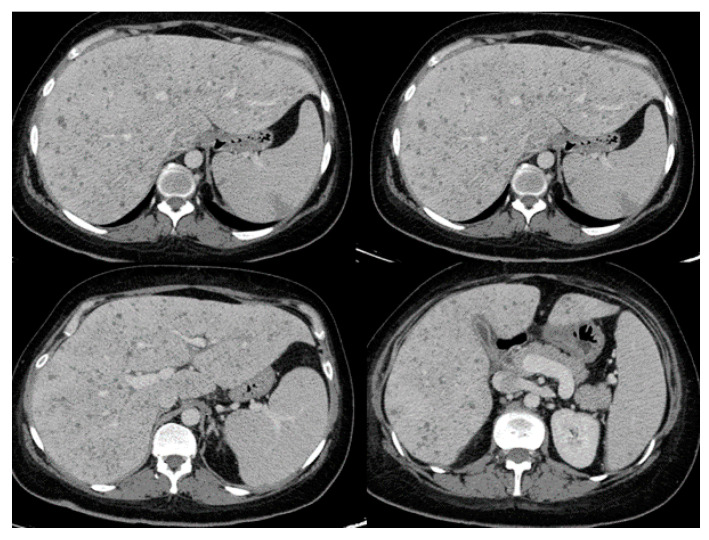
Abdominal computed tomography showing hepatosplenic abscesses in a patient with invasive *Saprochaete clavata* infection (reproduced from Del Principe, M.I et al. Mycoses 2016, 59, 594–601, doi:10.1111/myc.12508 with permission from John Wiley and Sons under license number 4915460075071).
